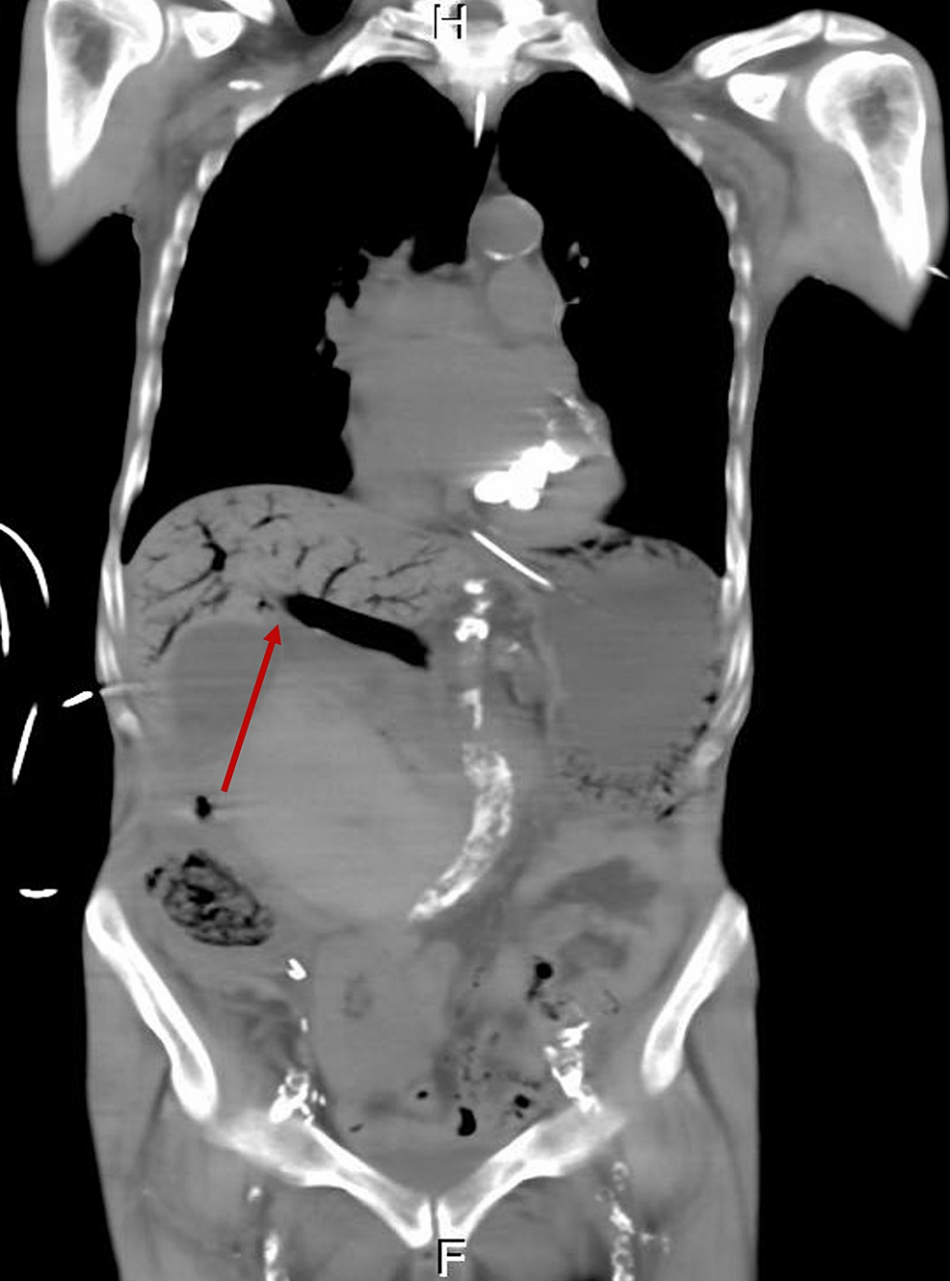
CT Abdomen/Pelvis (coronal view) The image is showing HPVG (Hepatic Portal Venous Gas)
